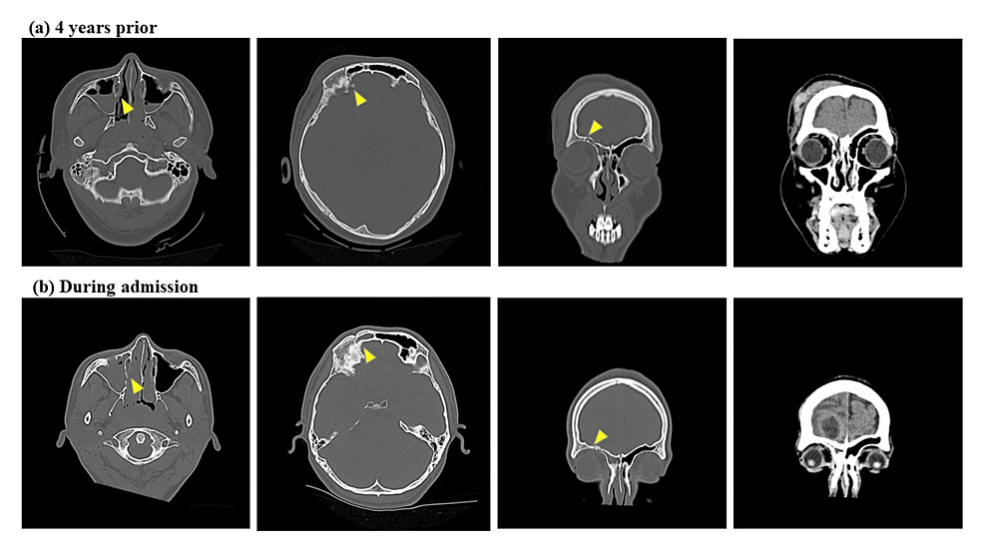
CT images of the frontal skull fracture four years prior and the brain abscess during this admission. (a) CT following a vehicular accident four years prior, showing a skull fracture in the right frontal and maxillary sinus. (b) CT taken during this admission due to a brain abscess, revealing incomplete fusion of the previous skull fracture in the right frontal and maxillary sinus.
